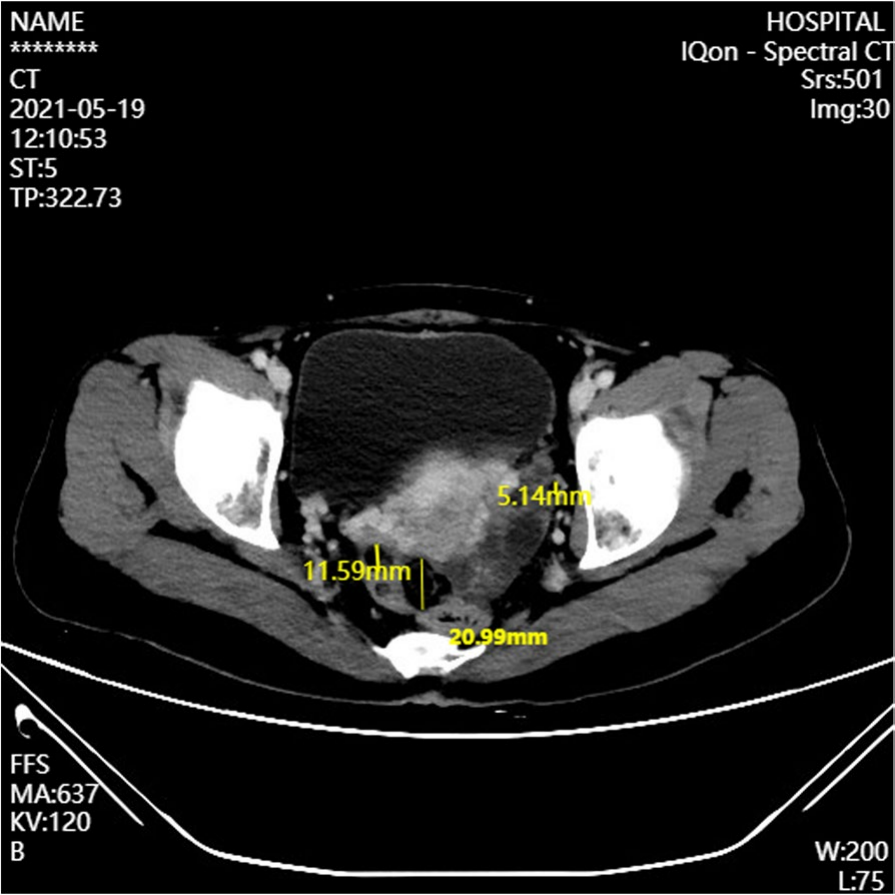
Pelvic CT revealed new-onset lesions in the appendages on both sides
